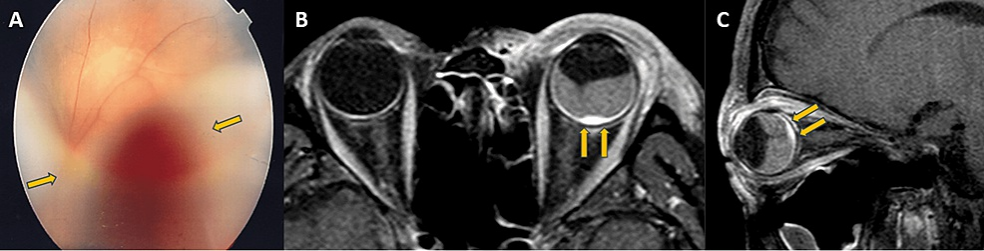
Color fundus photography (A) and contrast-enhanced MRI (B, C) of circumscribed choroidal hemangioma before proton beam therapy. Color fundus photography (A) showed retinal detachment from the optic disc to the macula  (arrows in A). Contrast-enhanced MRI (B, C) showed extensive retinal detachment and 10×4 mm hemangioma in the left eye (arrows in B, C).
